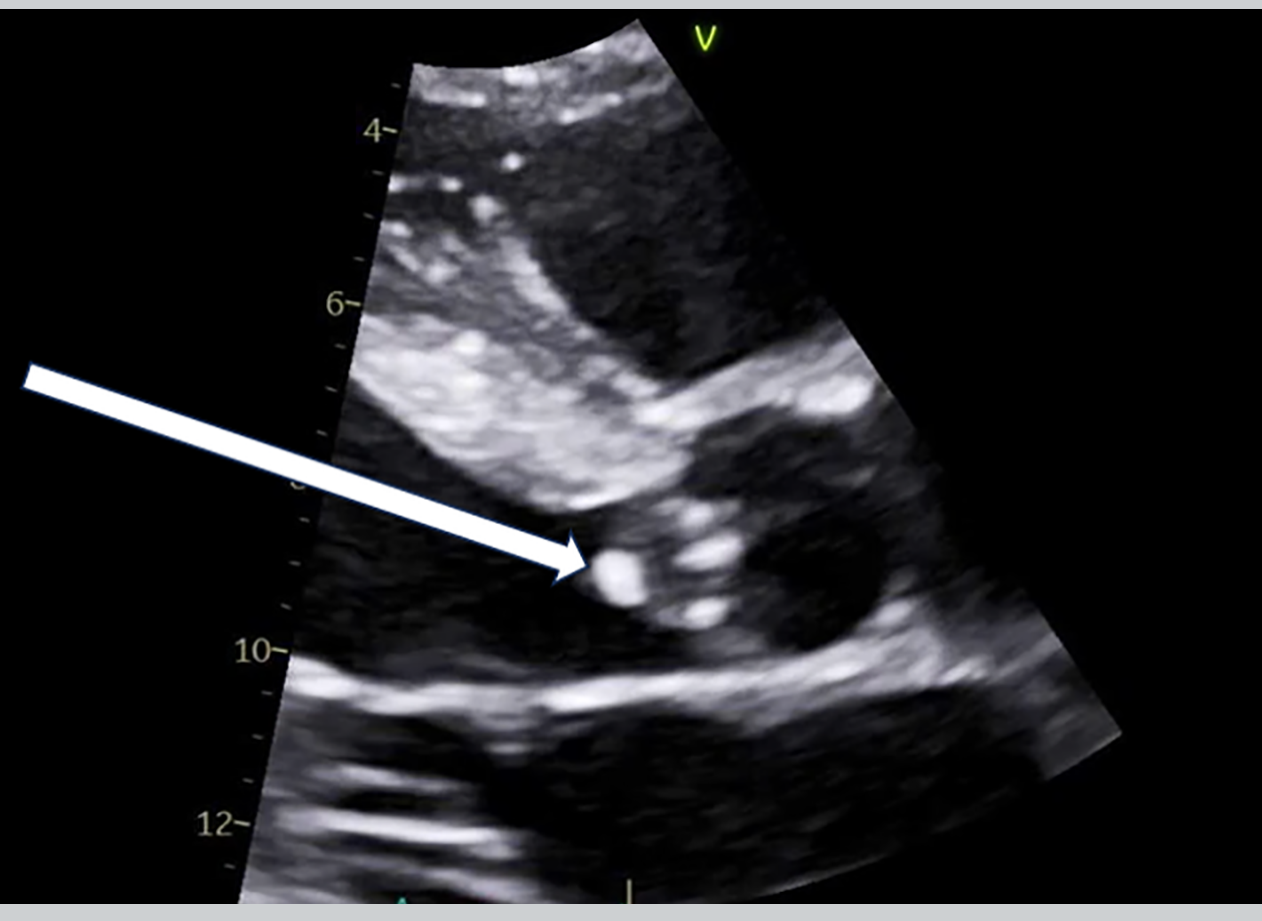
Transthoracic echocardiography (TTE) parasternal long axis zoomed view at the left ventricular outflow tract confirming the left coronary cusp vegetation pointed by the arrow.
